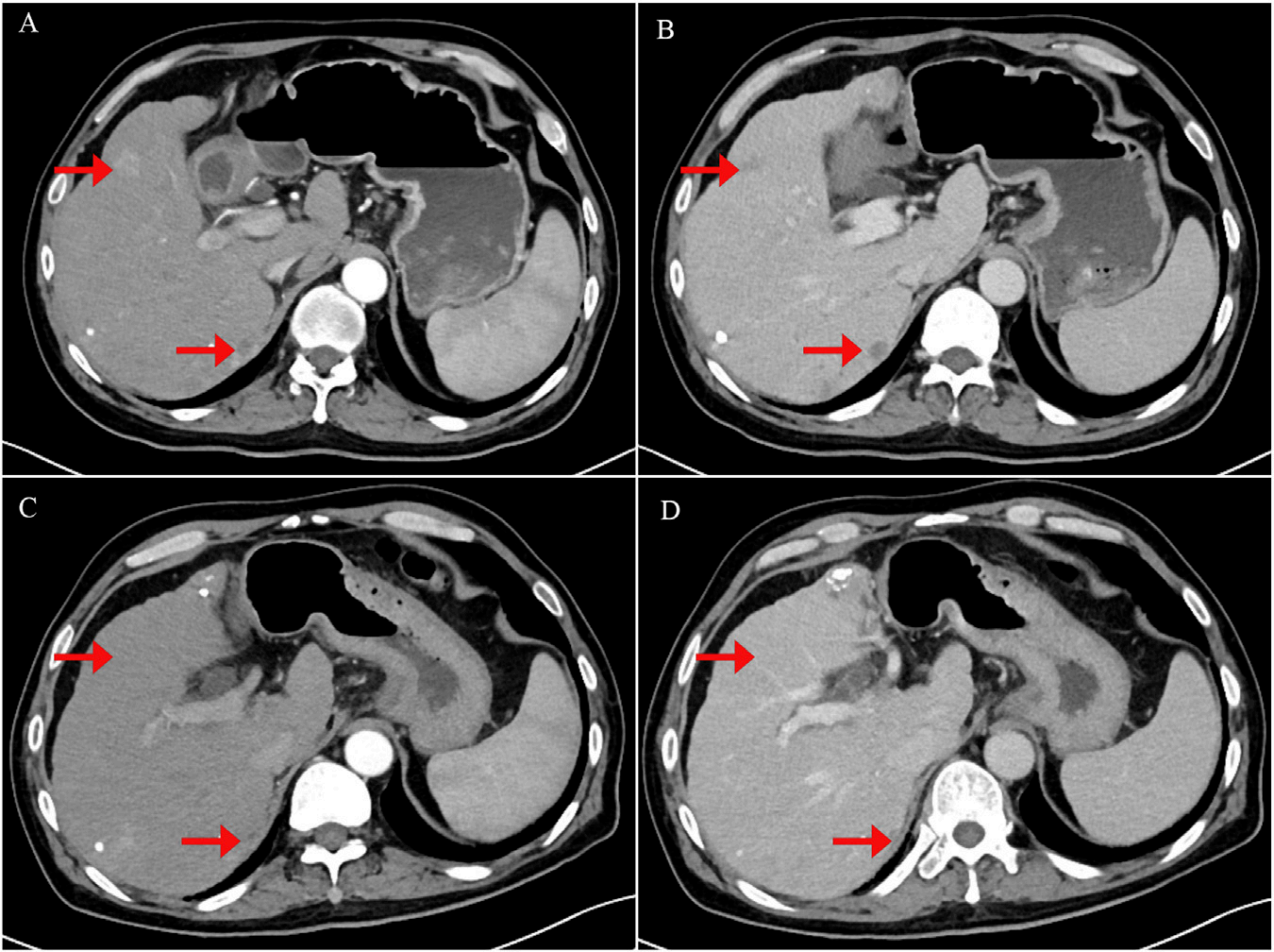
Contrast-enhanced abdominal computerized tomography (CT) scan images showing tumor recurrence and treatment response following crizotinib therapy. (A) Arterial phase image demonstrating a strongly enhancing nodule consistent with tumor recurrence. (B) Portal venous phase image showing persistent but slightly decreased enhancement of the lesion. (C) Arterial phase image obtained 1 month after initiation of crizotinib therapy, showing tumor shrinkage and reduced arterial enhancement of the nodule. (D) Portal venous phase post-treatment image demonstrating further tumor shrinkage with near-complete resolution of nodule enhancement.
